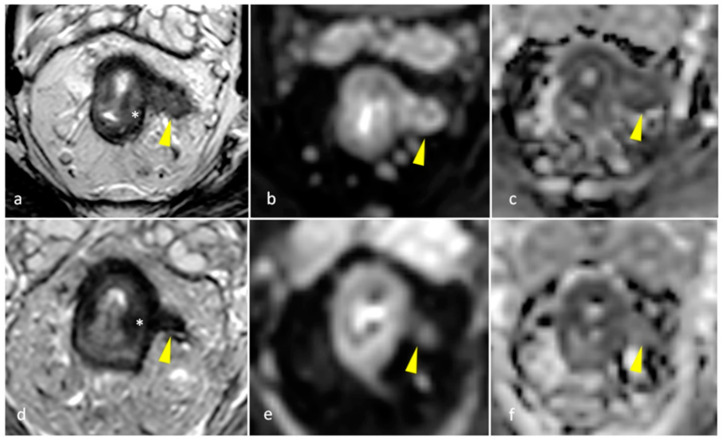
Pretreatment (a) axial T2-weighted TSE showing EMVI (arrow) along the left aspect of a rectal mass (*) as an intermediate-signal-intensity lobulated, elongated structure showing high signal on (b) DWI and restricted diffusion (low signal) on (c) ADC map. Post-treatment images show decreased signal of the rectal mass (*) and thinning of EMVI (arrow), appearing as a low signal intensity fibrotic band on (d) T2-weighted TSE, with a faint high-signal-intensity structure on (e) DWI, with no restricted diffusion (high signal) on (f) ADC map, suggesting replacement of tumoral tissue with fibrosis (mrTRG 2).
